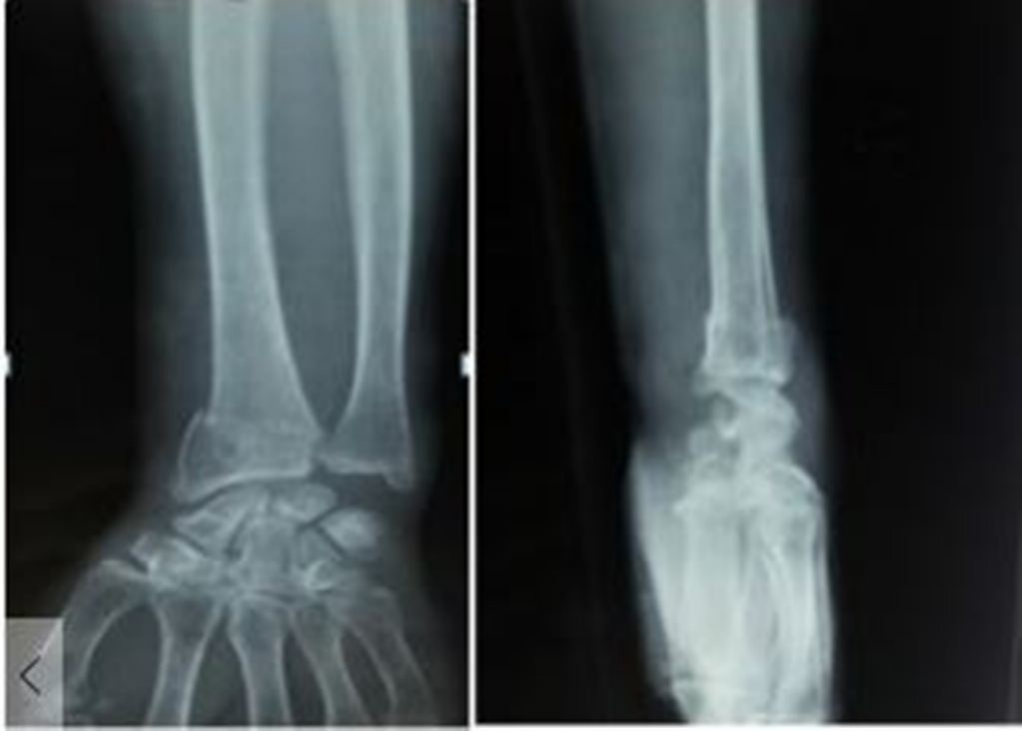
Radiographs anteroposterior and lateral view showing fracture of distal radius
